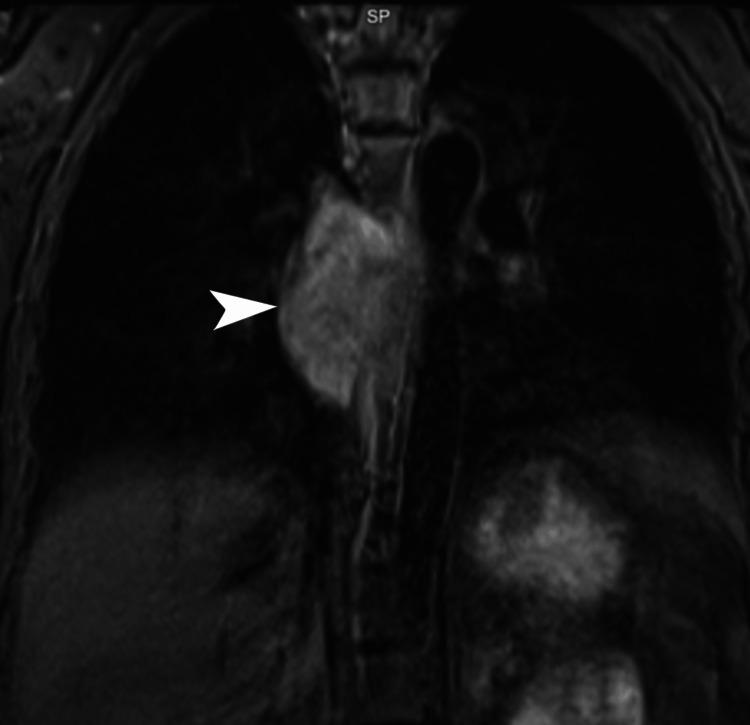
MRI of the subcarinal nodal mass. Coronal T2-weighted MRI images of the chest demonstrate a T2-hyperintense nodal mass in the subcarinal region with bilateral extension (white arrowhead). The lesion is seen producing extrinsic compression of the thoracic esophagus and extending superiorly toward the aortopulmonary region. MRI: magnetic resonance imaging
